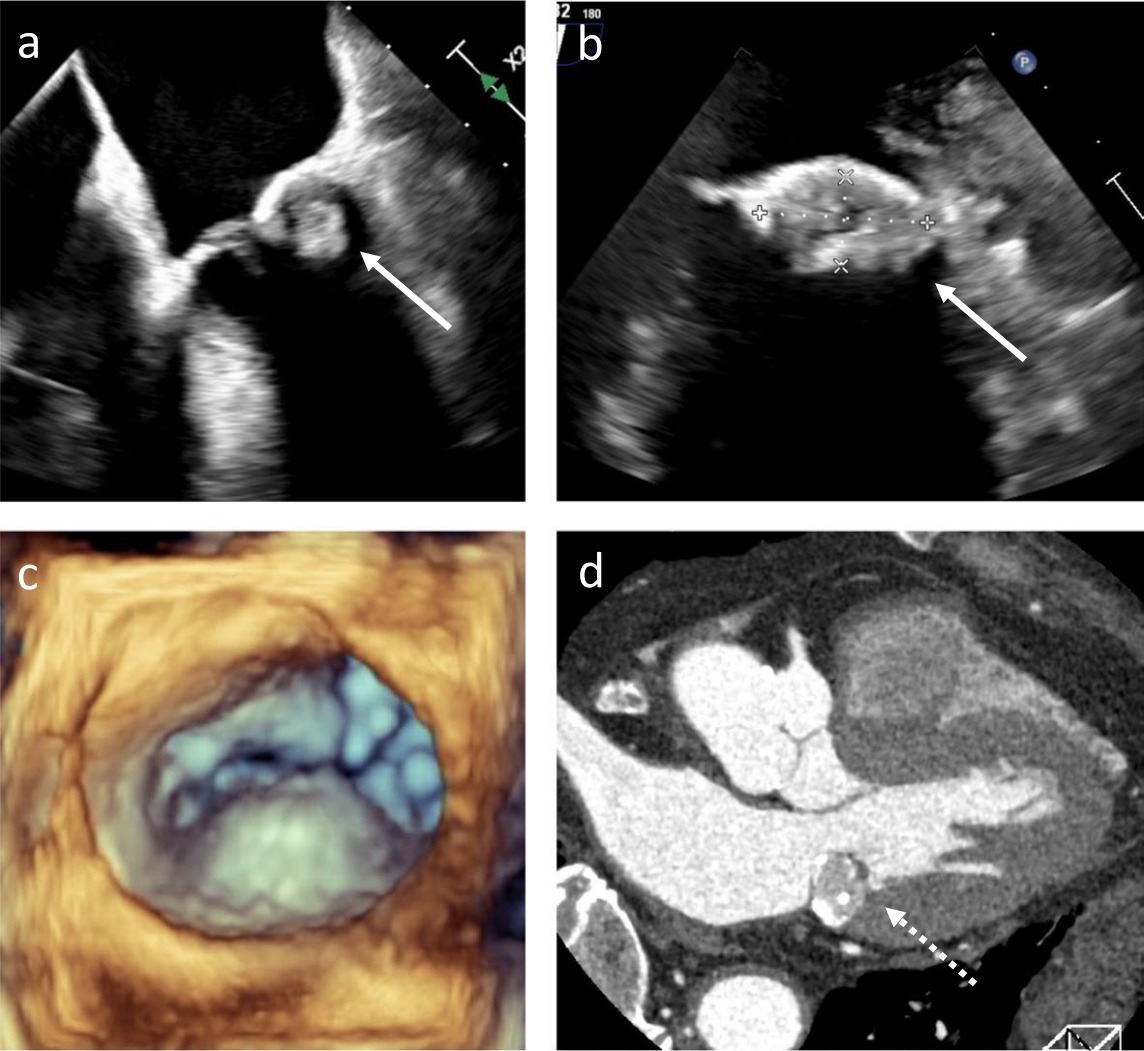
Preoperative examinational findings. a, b Transesophageal echocardiography. The white arrow indicates a posterior leaflet tumor that rose from the left ventricular side with a 12 × 22-mm diameter. c 3-dimensional transesophageal echocardiography shows a bulging of the middle posterior leaflet. d Cardiac computed tomography angiography. A white broken arrow indicates a calcified amorphous tumor that invaded the mitral valve annulus and left ventricular muscle
